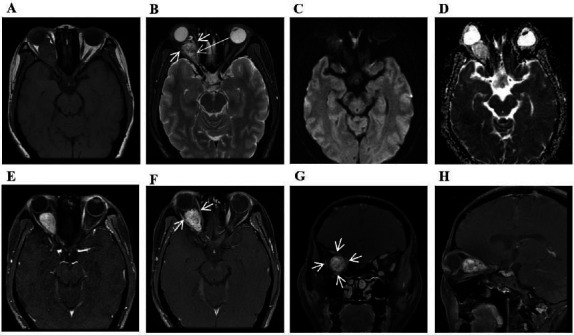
The mass showed isointensity on T_1_WI (A), heterogeneous hyperintensity with multiple flow voids (long arrow) on FS-T_2_WI (B), hypointensity on DWI (C), and hyperintensity on the ADC map (D). Heterogeneous enhancement was demonstrated on DCE-MRI (E) and Gd- FS-T_1_WI (F-H). A dilated vein was identified in the left anterior aspect of the mass (B, E). It should be noted that the superior, inferior, medial, and lateral rectus muscles of the right eye (short arrows) distinctly atrophied with a lower signal intensity on FS-T_2_WI (B) and less apparent enhancement than the left normal ones (F, G).
